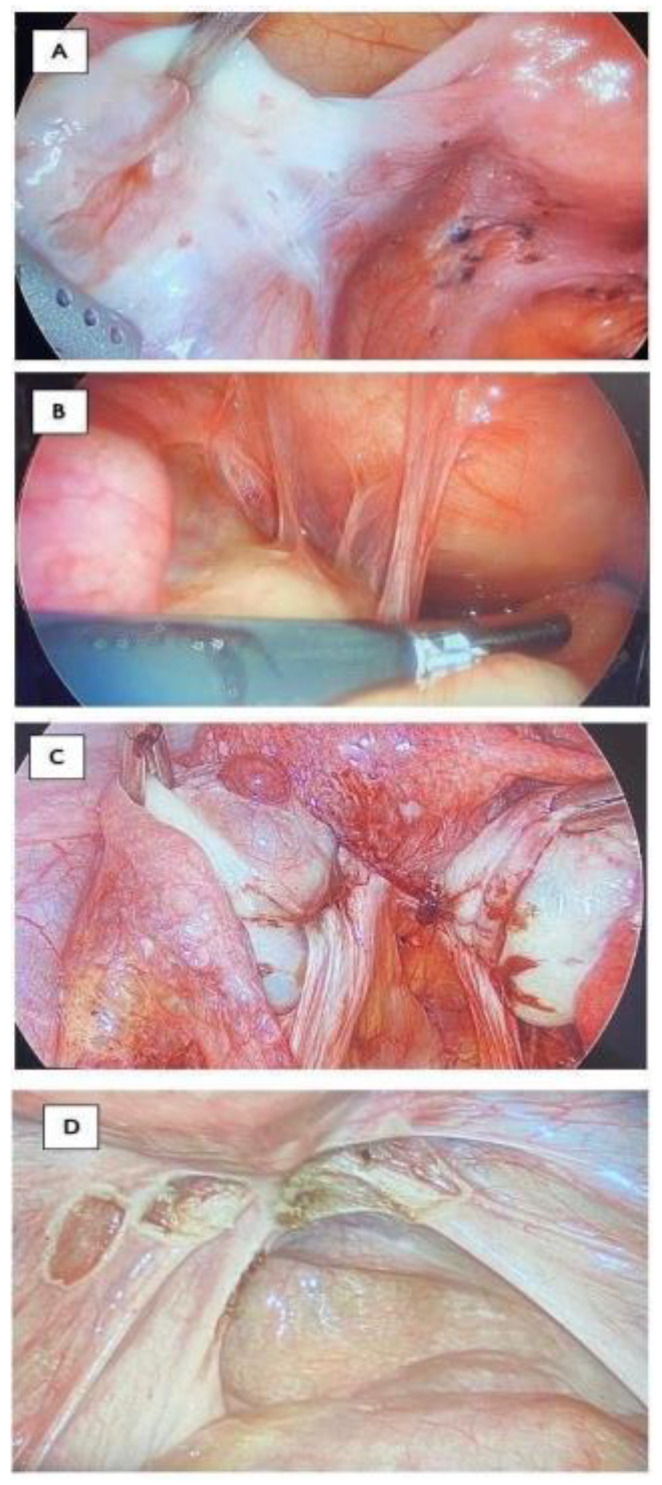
(A) Endometriosis of serosa and subserosa of the left ureter in a patient with unexplained infertility, with failed IVF and IUI cycles. (B) Filmy peritubal and periovarian adhesions in a patient with normal HSG. (C) A case of severe endometriosis with normal HSG and imaging and transvaginal ultrasound. (D) Suspicious lesions for endometriosis have been biopsied.
